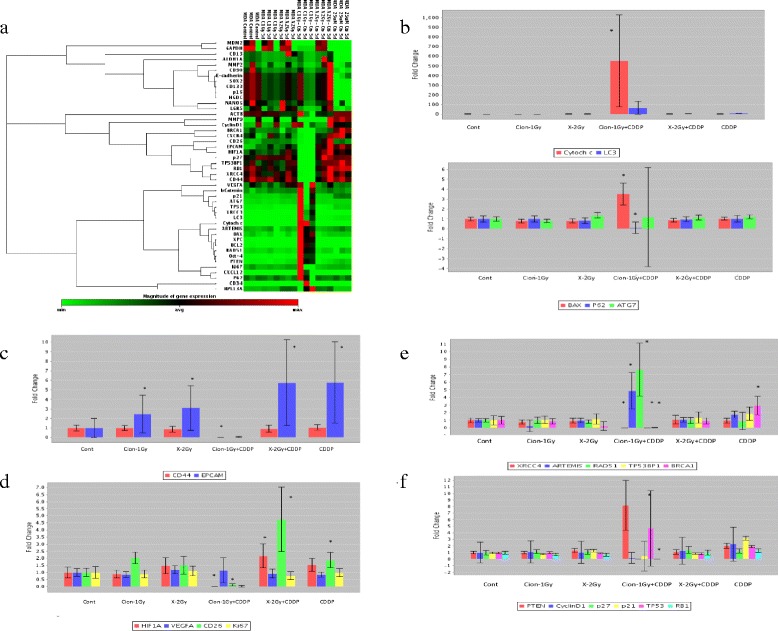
a. Clustergram of Custom RT-PCR Array of CSCs delivered from MDA-MB-231 cells 5 days after a carbon ion beam, X-ray alone or in combination with 25 μM of cisplatin (CDDP). CDDP was added prior to irradiation and treated for 5 days. b. Expression of apoptosis- and autophagy-related genes after carbon ion beam, X-ray alone or in combination with CDDP in CSCs. c. Expression CSC markers after carbon ion beam, X-ray alone or in combination with CDDP in CSCs. d. Expression of angiogenesis-, metastasis-related genes after carbon ion beam, X-ray alone or in combination with CDDP in CSCs. e. Expression of DNA repair-related genes after carbon ion beam, X-ray alone or in combination with CDDP in CSCs. f. Expression of cell cycle-related genes after carbon ion beam, X-ray alone or in combination with CDDP in CSCs. *, *p* < 0.01, compared to control. All experiments were performed in triplicate (*n* = 3)
